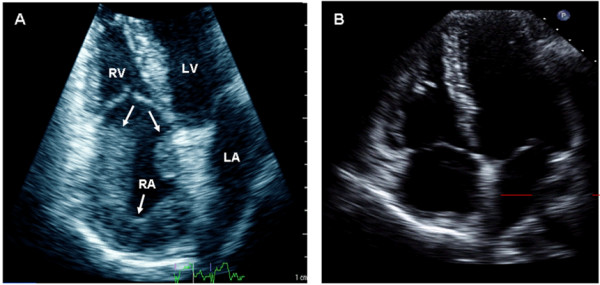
Two-dimensional echocardiography showed the large right atrial (RA) mass (arrows) on apical 4-chambers view (A) and almost complete resolution after the treatment (B).
